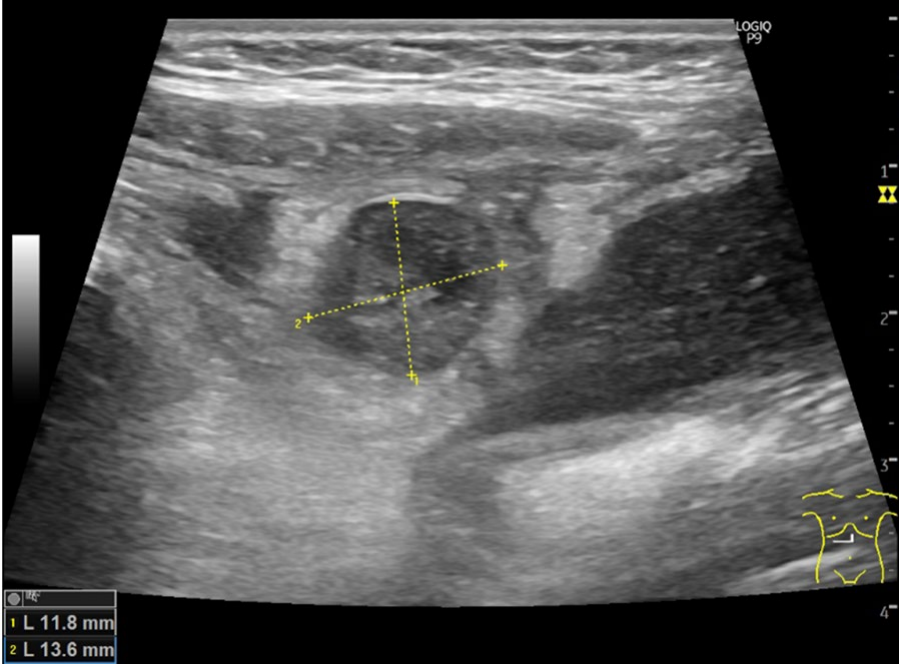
Abdominal ultrasonography with color Doppler imaging. A nonvascular lesion of mixed echogenicity was recognized near the round ligament of the liver
